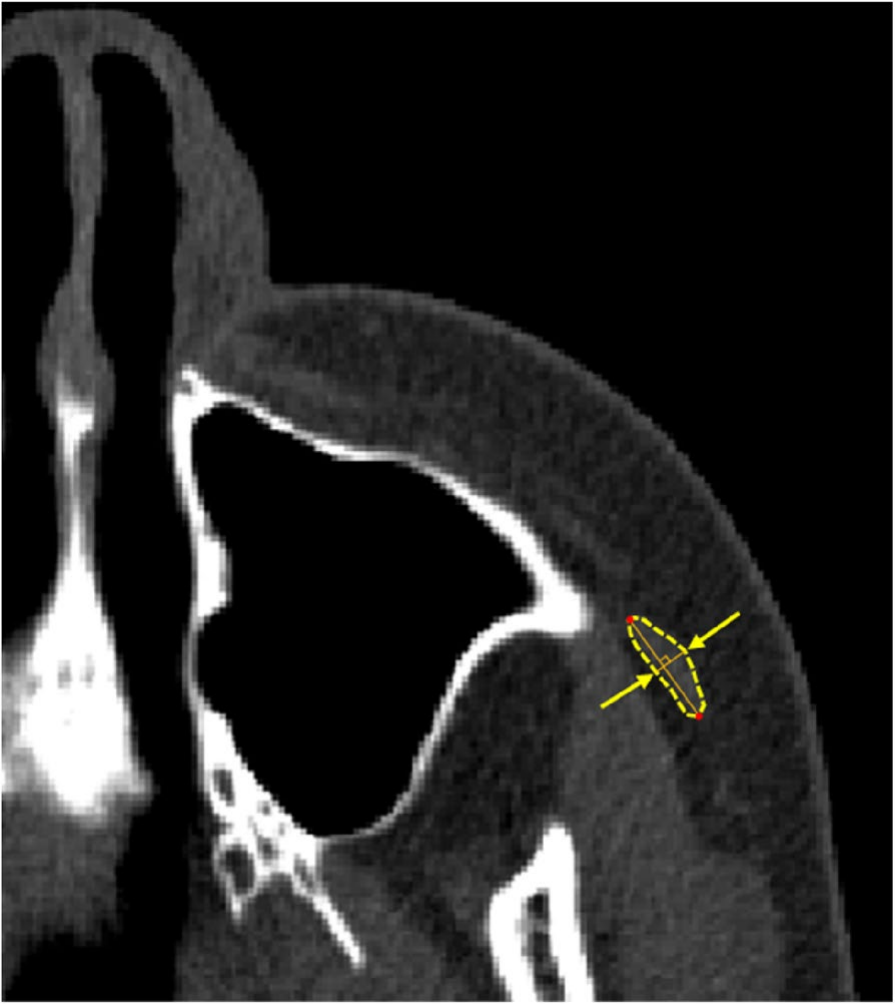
CT measurement for the zygomaticus major muscle. (1) Zygomaticus major muscle width. (2) CT value of the zygomaticus major muscle. CT, computed tomography
